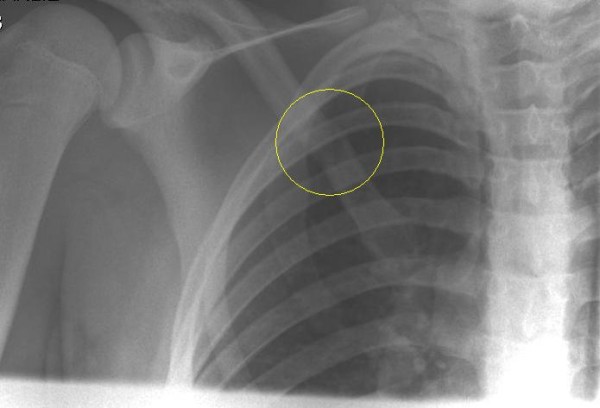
AP X-Ray AP X-Ray showing an undisplaced fracture.
